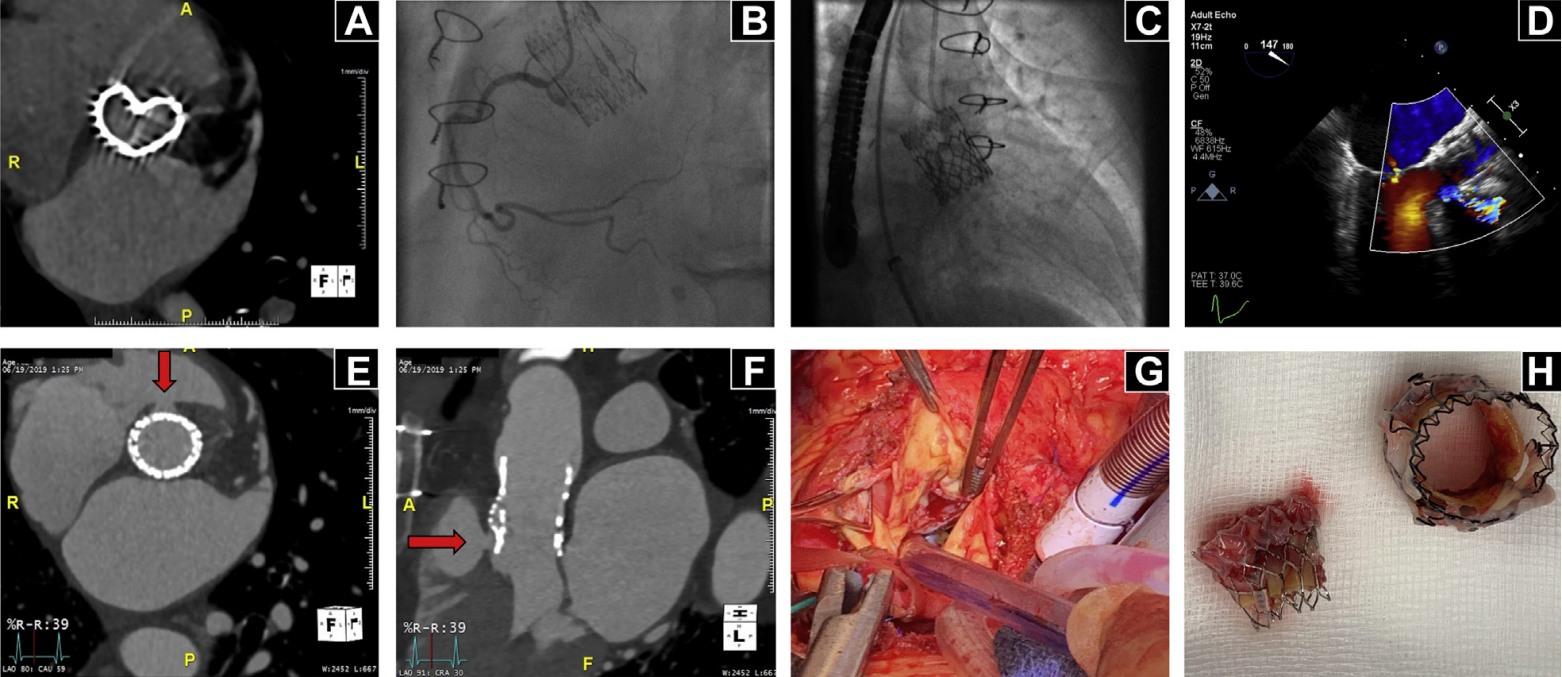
CT Demonstrates Stent Infolding, Distortion, and Collapse of Perceval Sutureless Valve (A) CT imaging demonstrates stent infolding, distortion, and collapse of Perceval Sutureless valve. (B) Left anterior oblique view of a Perceval Sutureless valve during right coronary angiography. (C) Orthogonal view of a successful Sapien S3 valve-in-valve implant. (D) Mid-esophageal long-axis view on TEE demonstrates a fistulous connection (white arrow shows color Doppler flow) between the right coronary cusp and right ventricle. Axial (E) CT imaging of well-deployed valve-in-valve with fistulous connection (white arrow) confirmed by contrast leak (F). This is also demonstrated by 3-dimensional CT reconstruction (white arrow). (G) Resternotomy for surgical AVR. (H) Sapien 3 (left) and Perceval Sutureless (right) valves retrieved perioperatively. AVR = aortic valve replacement; CT = computed tomography; TEE = transesophageal echocardiography.
